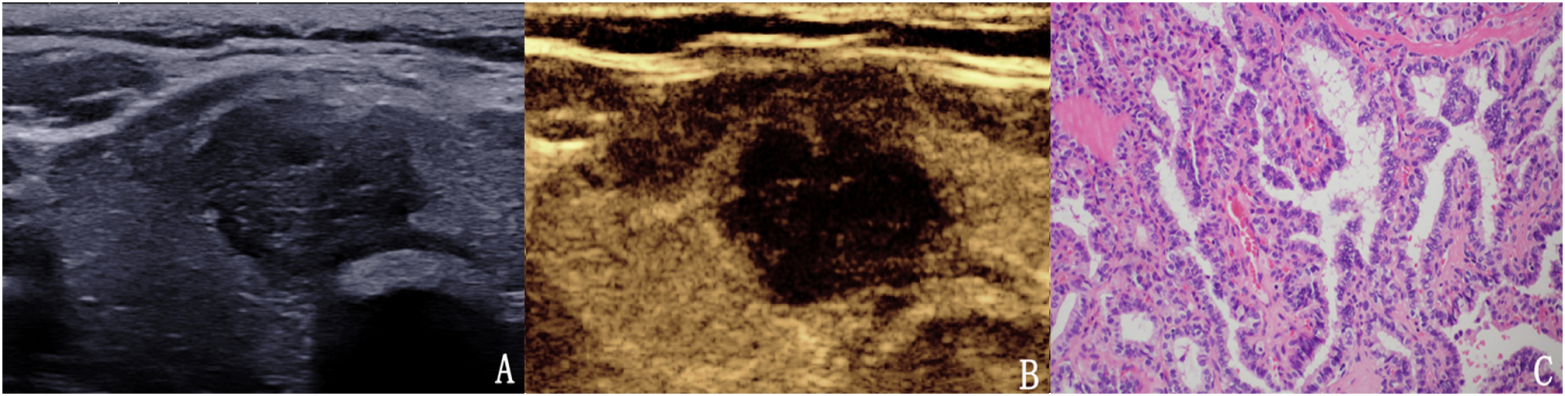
The case of a 51-year-old woman with a 20.4 × 15.1 × 16.9 mm solid mark hypo-echoic nodule in the right lobe of the thyroid. (A) Conventional two-dimensional image showing that the nodule had three malignant indicators (solid, mark hypo-echoic and irregular margin) and was classified with a TI-RADS score of 4b. (B) Ultrasound contrast image showing low enhancement. The modified version of the TI-RADS combined with CEUS returned a score of 5, and the diagnosis indicated a malignant nodule. (C) Pathological image of the lesion, a PTC.
